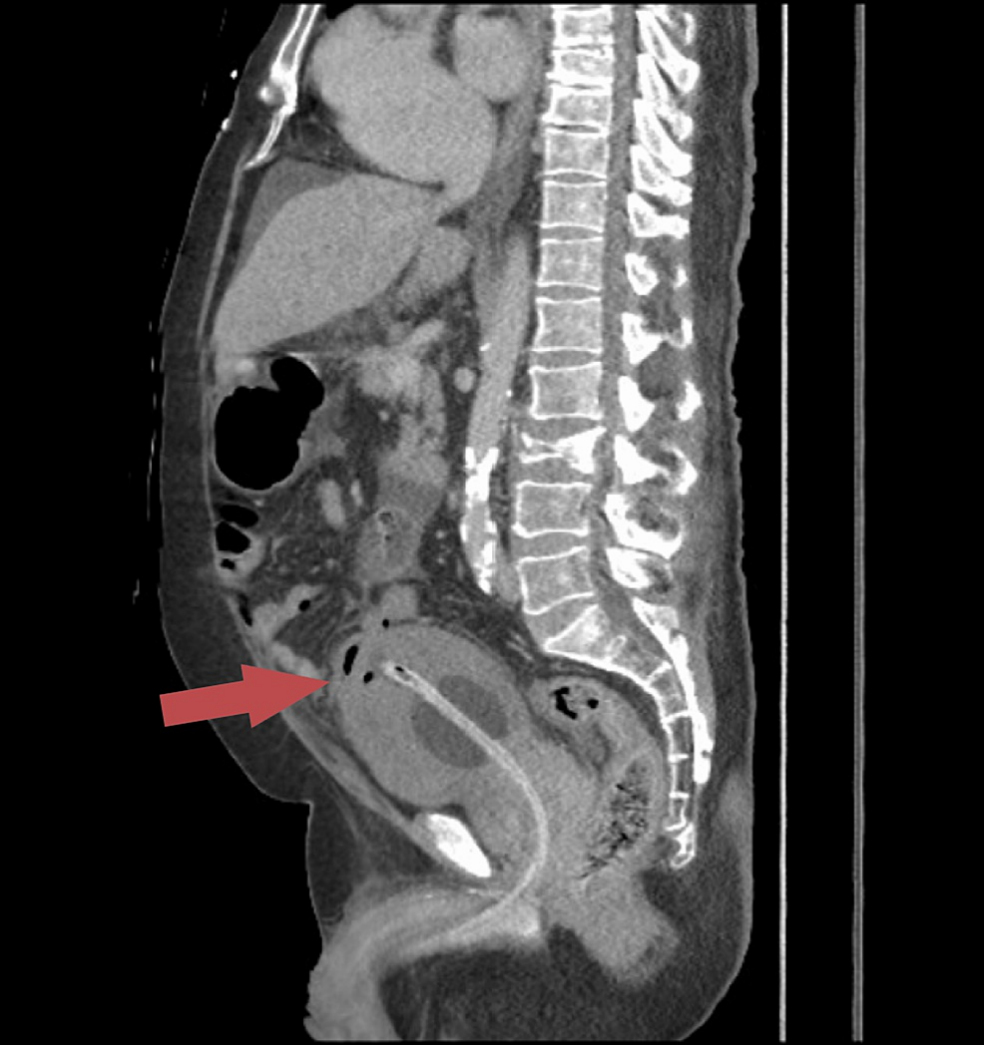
CT of the kidney, ureter, and bladder (sagittal view) showing emphysematous cystitis complicated with bladder rupture and large high density intra-abdominal collection
